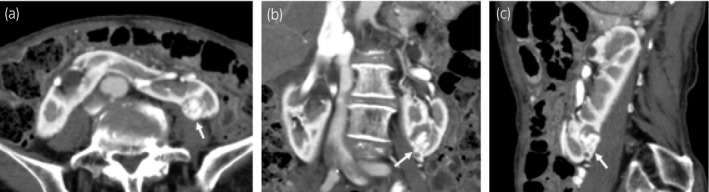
Computed tomography revealed a 2‐cm tumor (white arrow) in the left posterior aspect of the lower pole of the horseshoe kidney. (a) axial image; (b) coronal image; (c) sagittal image
